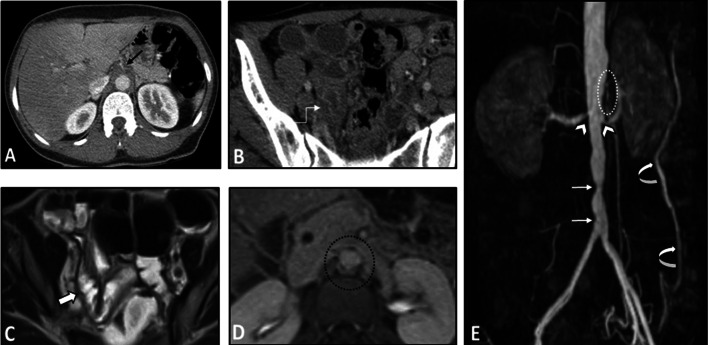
Intestinal vasculitis in a 35-year-old female with known Takayasu arteritis presenting with RLQ pain and elevated ESR. Axial contrast-enhanced CT scan (A) shows mural thickening and severe stenosis at the origin of the celiac artery (thin black arrow). Axial CT image (B) demonstrates circumferential mural thickening and submucosal edema at a single distal ileal segment (elbow arrow). MRE (C) was done following corticosteroid therapy after six days. Axial T2-W image shows complete resolution of corresponding ileal involvement (thick white arrow). Axial post-contrast T1-W image (D) displays concentric and irregular mural thickening and enhancement of abdominal aorta (black dotted oval) with double rings appearance (inner low and outer high enhancement). MR angiography (E) was performed. MIP image shows irregularity and multi-segmental stenosis at the distal abdominal aorta (thin white arrows). There is also bilateral stenosis at the origin of renal arteries (white arrowheads). The proximal segment of SMA shows irregularity and severe stenosis (white dotted oval). Abnormal dilatation of the arc of Riolan (white curved arrows) is evident, which supplies collateral flow to the SMA territory
